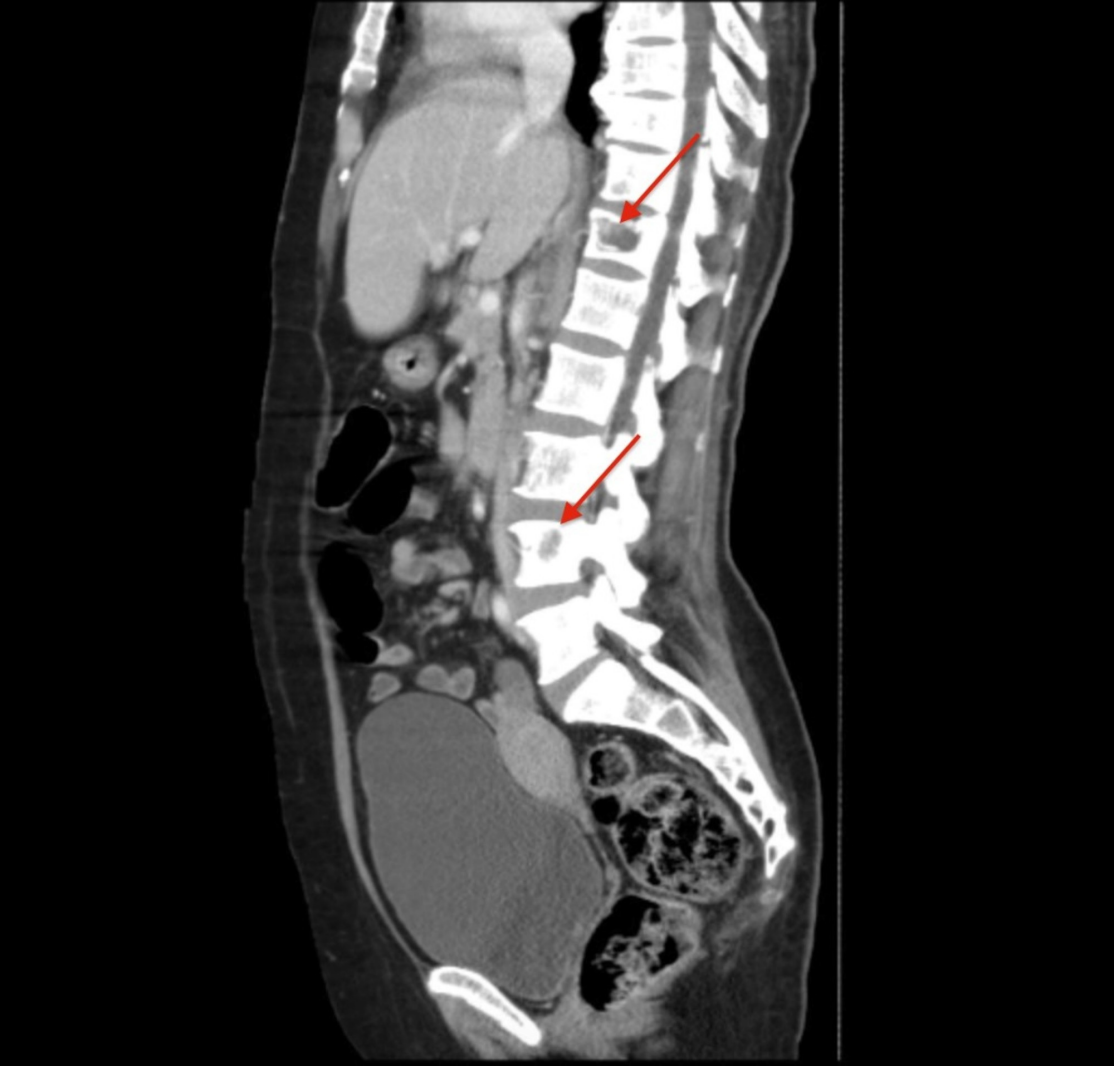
Sagittal section of a CT scan showing multiple lytic lesions in the thoracic spine and lumbar spine (red arrows) CT: computed tomography
